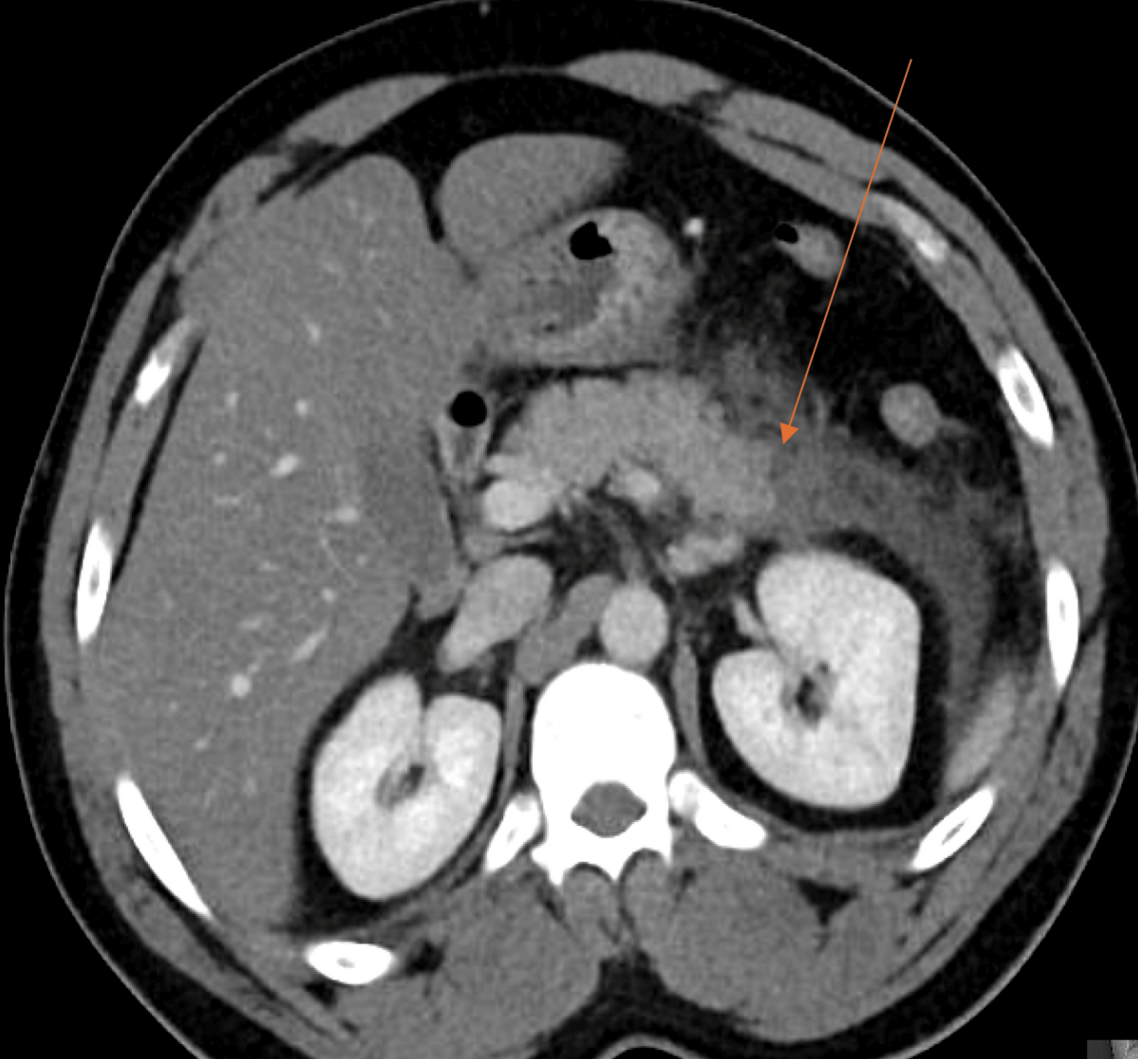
CT abdomen and pelvis showing acute interstitial edematous pancreatitis, obtained on admission, as indicated by the orange arrow CT: computed tomography
